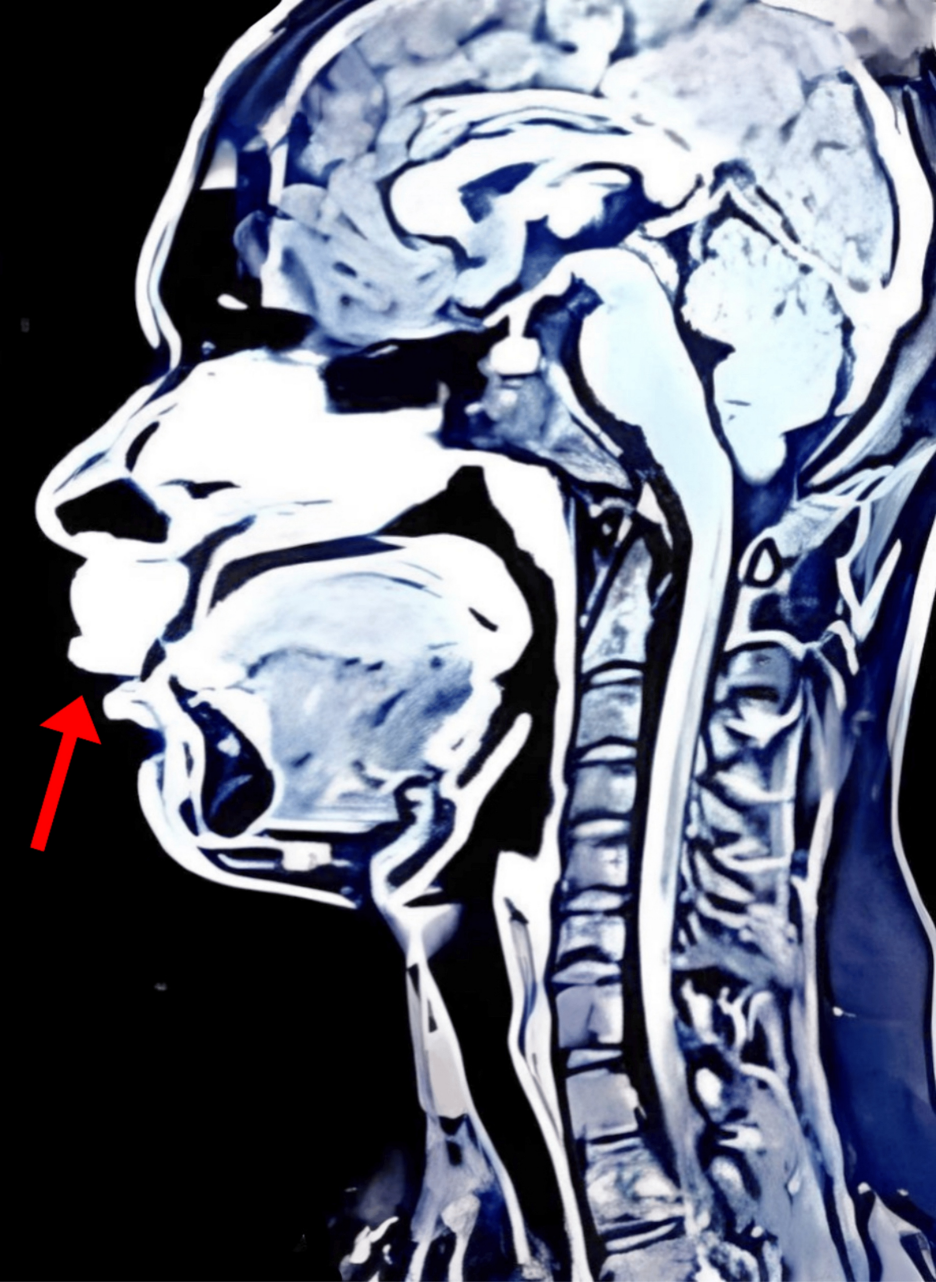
Preoperative sagittal magnetic resonance imaging (MRI)
